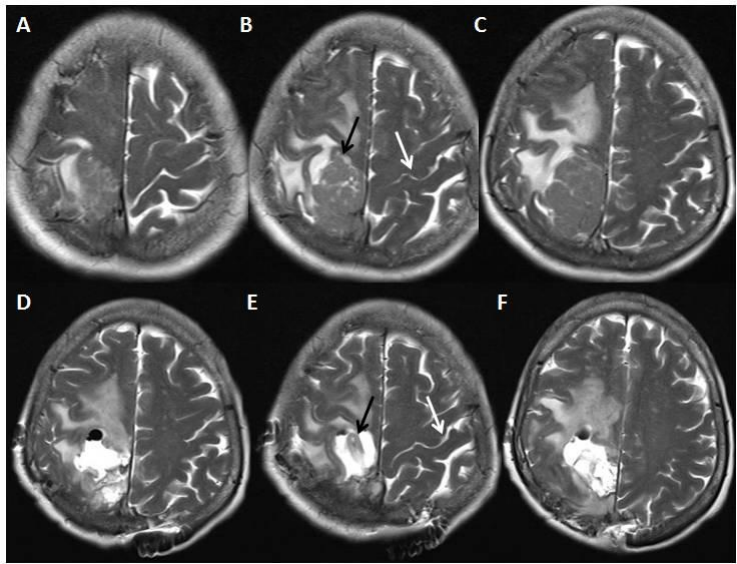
A, B, C (Table 1: Case 1, Grade 2 meningioma): Noncontrasted T2 weighted cranial Magnetic resonance image –it was taken in the preoperative period- scans showing hypointense mass lesion occupied the right motor cortex. Moreover, black arrow showing invasion of the right precentral gyrus (right motor cortex) and anterior part of the right parietal lobe. White arrow is showing the intact left precentral gyrus (motor cortex). D, E, F: Noncontrasted T2 weighted cranial Magnetic resonance image –it was taken in the postoperative period- scans showing hyperintense area at the region of the right precentral gyrus and anterior part of the parietal lobe. Black arrow shows this area, and on the left hemisphere, white arrow indicating left precentral gyrus (motor cortex)*.
